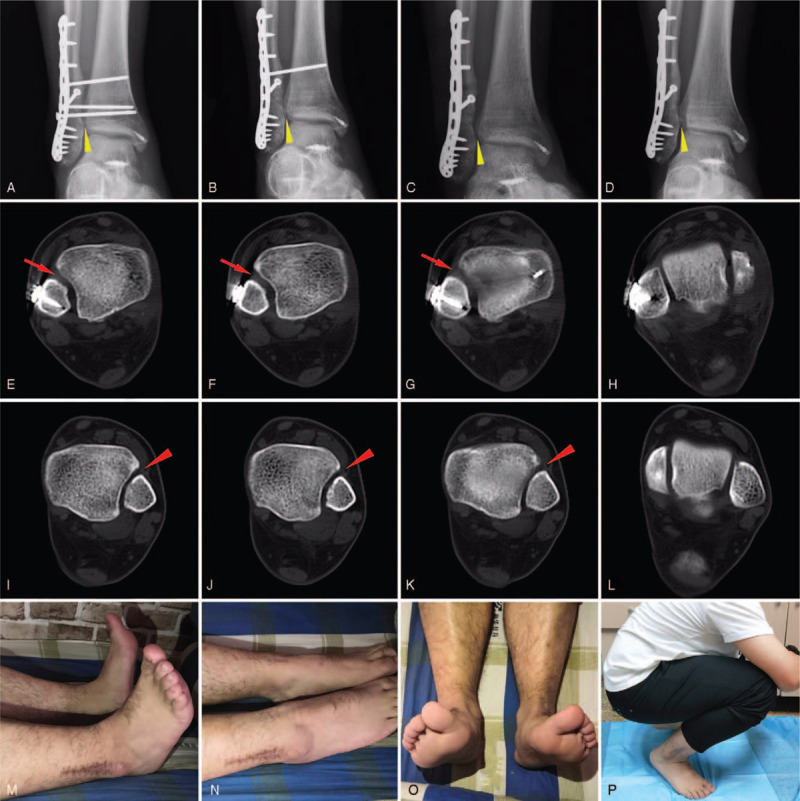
(A) 12-week post-operative X-ray image, (B) X-ray image after 2 syndesmosis screws were removed at 12-week. (C) X-ray image after all syndesmosis screws were removed at 16-week. (D) X-ray image at 1-year follow-up. After all screws removed, the syndesmosis was slightly increased (yellow triangle arrows). (E–L) the CT images at 1-year follow-up confirmed the increase of injured syndesmosis (red arrows) than contralateral side (red triangle arrows). (M–P) The functional outcomes of injured ankle at 1-year follow-up showed satisfied result, the ROM of dorsiflexion and plantarflexion was almost normal.
